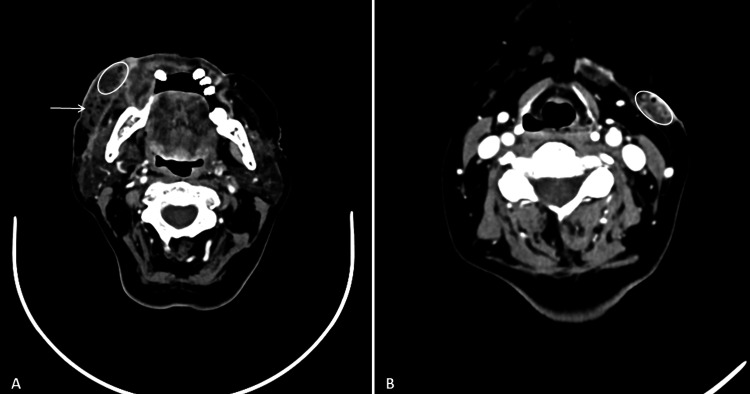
Axial contrast-enhanced CT scans: (A) The arrow indicates subcutaneous thickening and heterogeneity of the right hemiface with areas of subcutaneous gas. The oval contour highlights a hypodense collection with an internal gas bubble measuring 8.7 mm. (B) The oval contour marks a hypodense lesion within the hypodermis at the transition between the left submandibular and left cervical regions, containing small gas bubbles and measuring 17 × 8 mm, consistent with an abscess. CT: computed tomography
